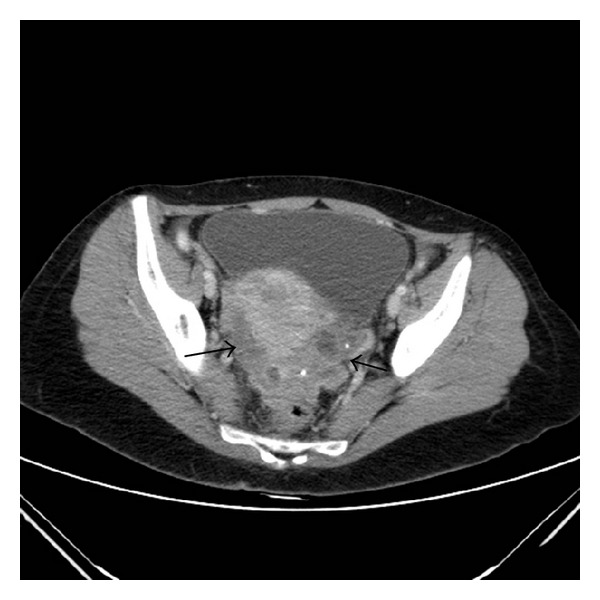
Axial CT image after contrast administration shows heterogeneous iso- to hypoattenuating masses in the pouch of Douglas. Inside we can see some punctiform calcifications.
